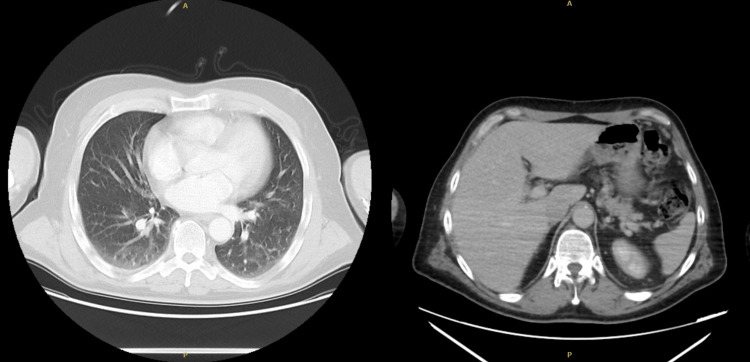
CT of the chest (left) and abdomen (right) showing normal lung parenchyma and no organomegaly. No signs of malignancy or abnormal enlargement are seen. CT: computed tomography
